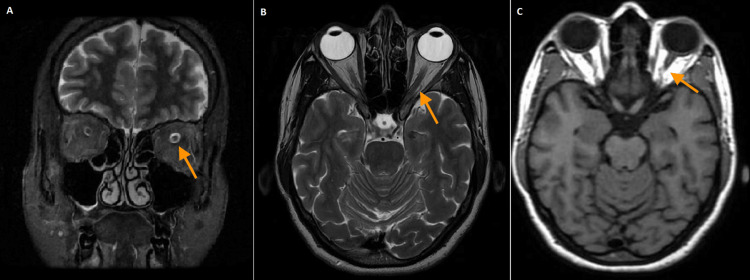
Brain and orbit MRI showing left optic nerve (orange arrows) hyperintensity in coronal STIR (A). Axial section T2 (B) and axial section T1 (C) demonstrate normal signal intensity of the left optic nerve MRI: magnetic resonance imaging; STIR: short tau inversion recovery sequence
